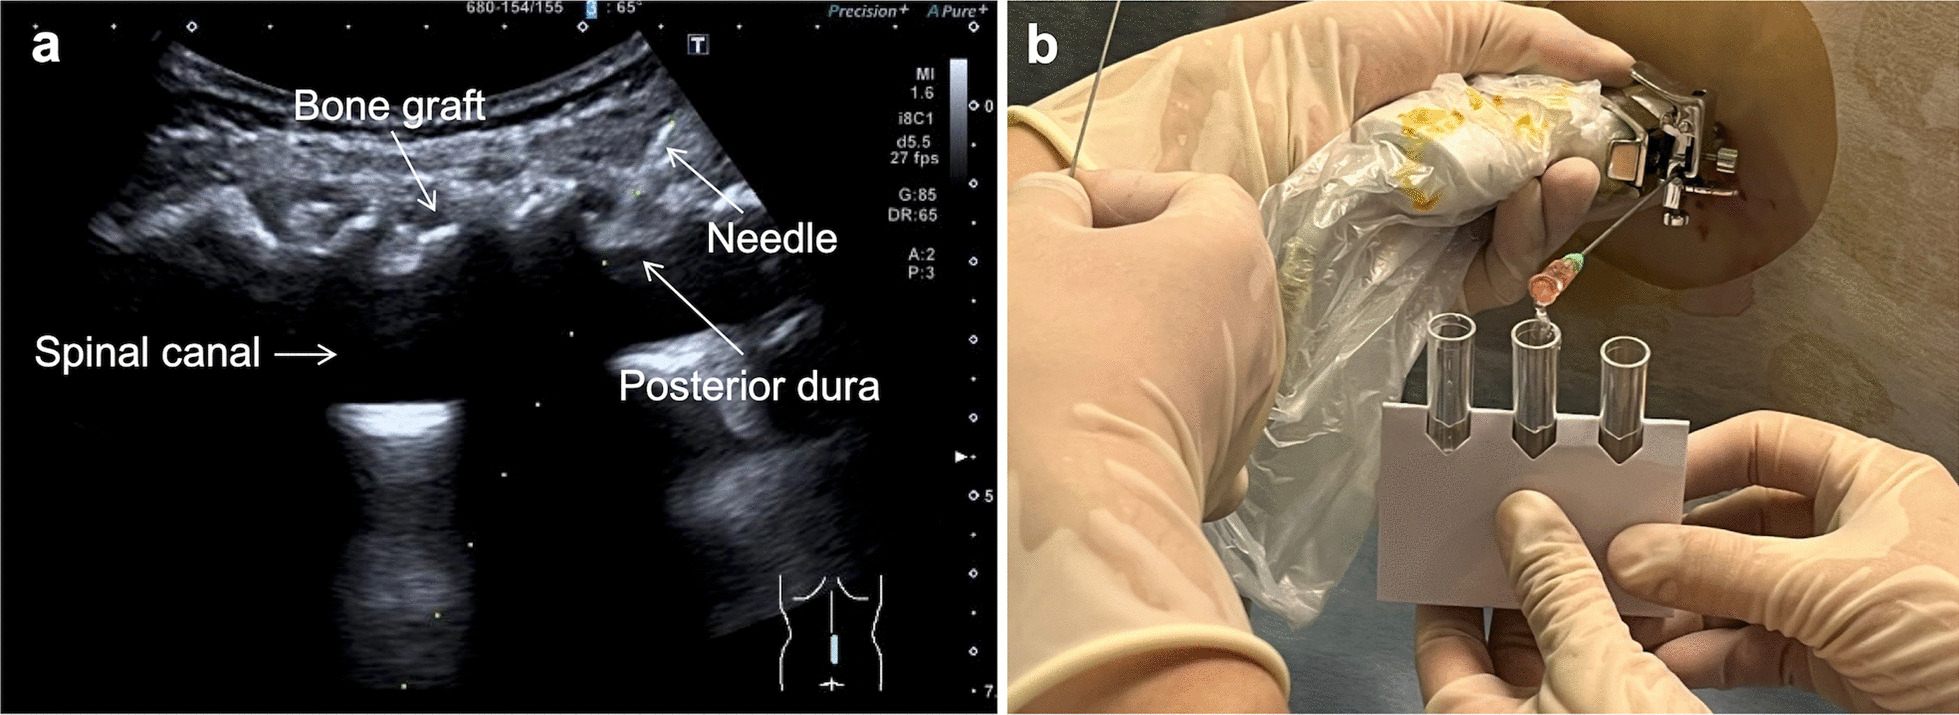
Real-time ultrasound-guided lumbar puncture in an SMA patient who had undergone spine fusion. a The ultrasound image showed that the needle reached the spinal canal in the intervertebral space reserved during spinal fusion. b Clear cerebrospinal fluid was successfully discharged from the puncture needle
